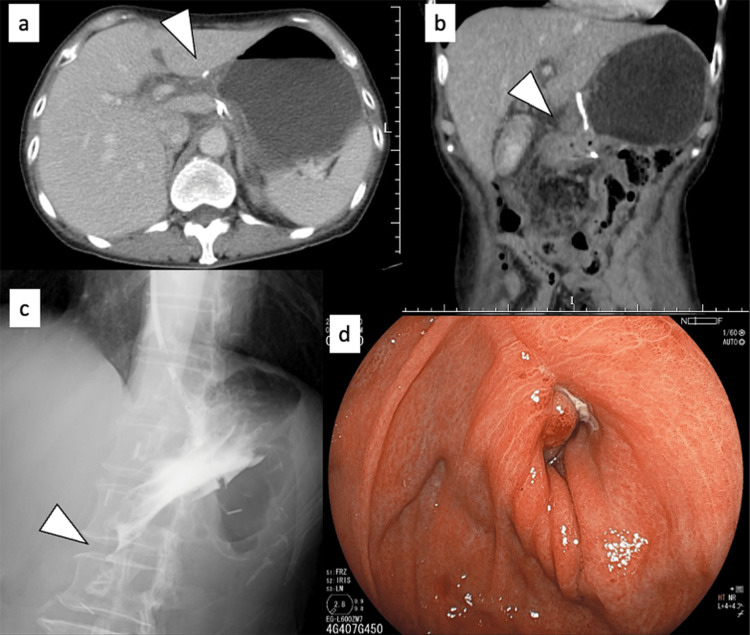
Images obtained before systemic steroid administration (between POD 11 and POD 13). The axial CT scan (a), coronal CT scan (b), and fluoroscopic examination (c) show that the gastroduodenal anastomosis exhibits edematous stenosis, and the remnant stomach is dilated because there is no gastric juice flow. The endoscopy (d) revealed that the duodenal mucosa is edematous and protruded into the lumen of the anastomosis. Arrowhead indicates the anastomosis. POD, postoperative day.
